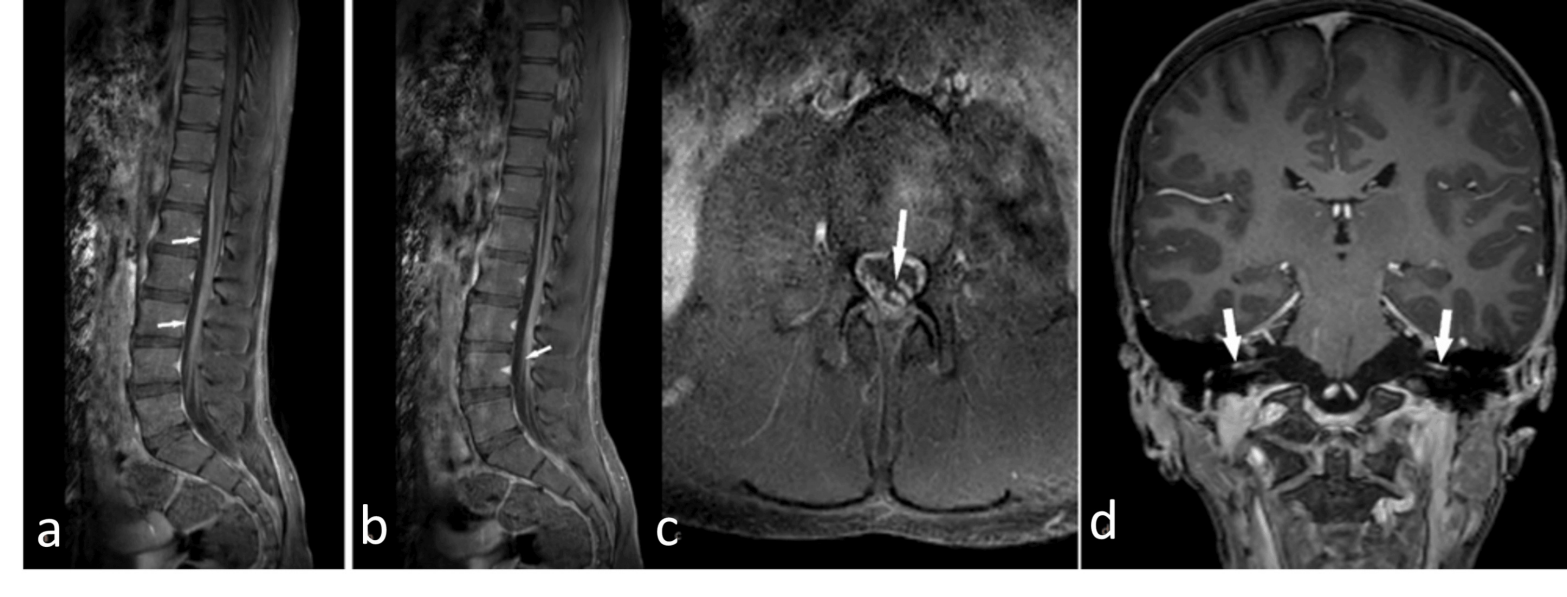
MRI examination of the patient at administration Contrast-enhanced MRI findings in cauda equina and bilateral facial neuritis Fat-suppressed, contrast-enhanced sagittal T1-weighted lumbar MRI shows marked contrast enhancement of thickened nerve roots of anterior (a) and posterior (b) cauda equina (white arrows). Fat-suppressed axial T1-weighted MRI shows marked contrast enhancement of thickened nerve roots of the cauda equina (c). Contrast-enhanced coronal T1-weighted brain MRI shows enhancement consistent with bilateral facial neuritis within the bilateral internal acoustic canals (d).
